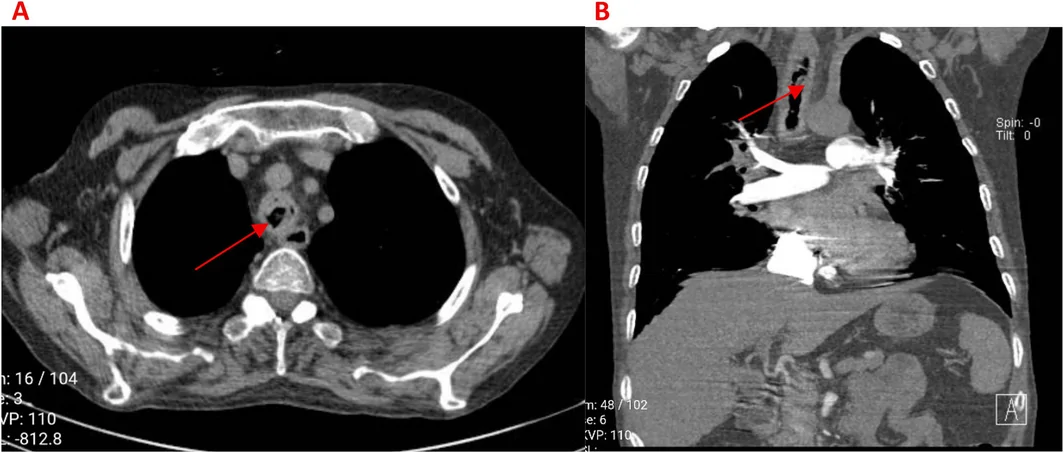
Coronal and axial chest CT images showing an intratracheal mass‐like lesion at the level just inferior to the suprasternal notch, with no evidence of associated pulmonary parenchymal involvement.
